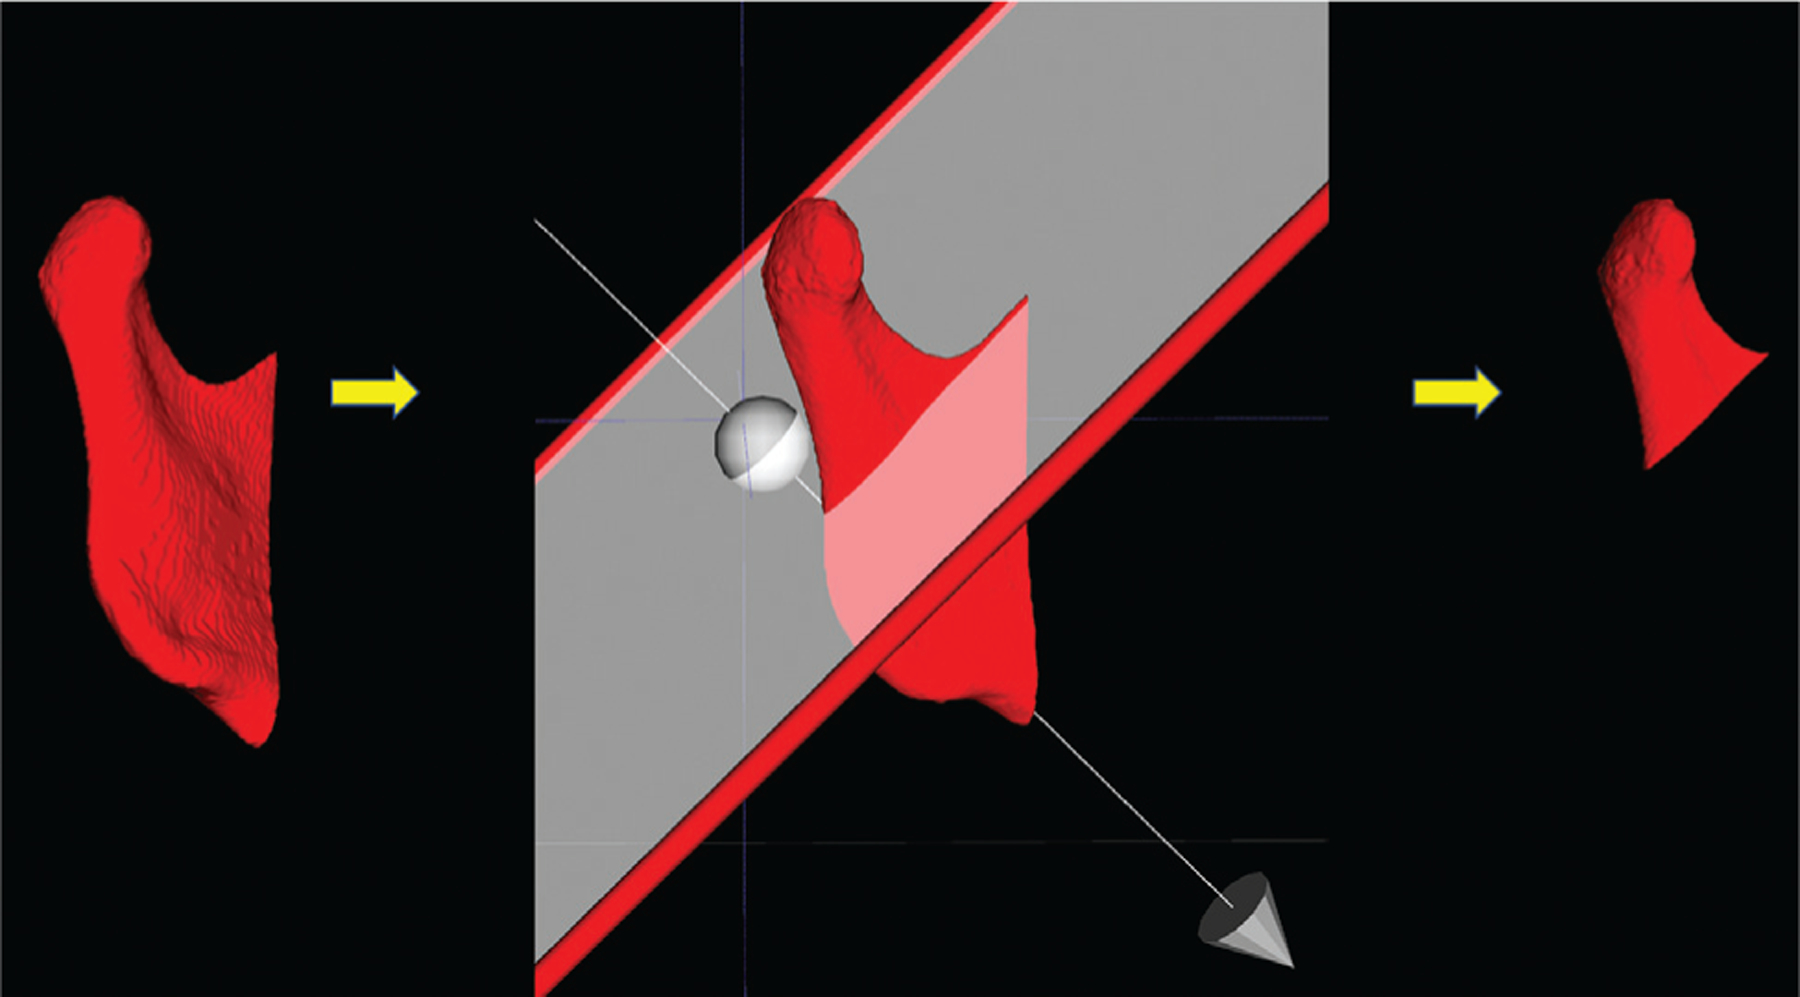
Segmentation of the condyles of the multispiral computed tomography (CT) and cone-beam computed tomography (CBCT) images at the T0 and T1 stages performed using the ITK-SNAP software. Segmented condyles were cut using the scalpel tool (ITK-SNAP software) to obtain condylar head for further assessment.
